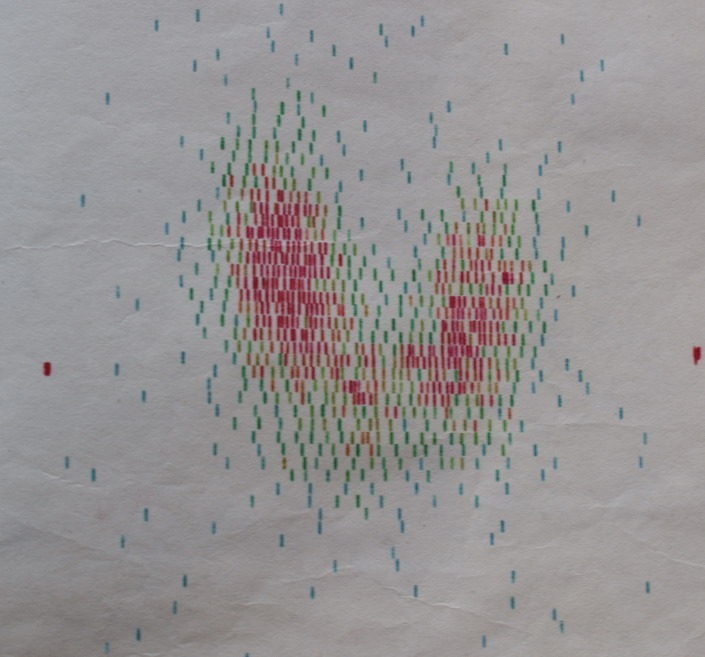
The I131 scintigraphy showing a large, homogenous thyroid gland
